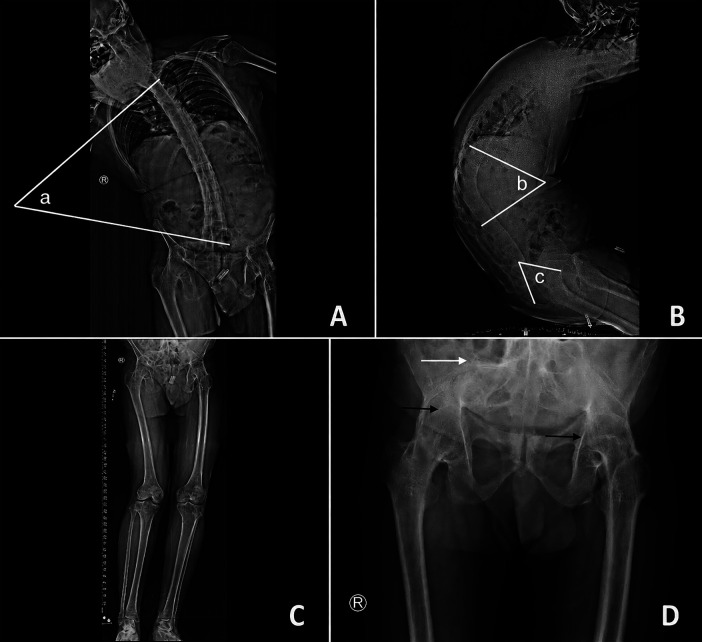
Preoperative x-ray films showed (A) bamboo-like changes in the vertebral bodies in the coronal plane of the spine, with the spine bending to the right, cobb angle of a = 50°; (B) kyphosis in the sagittal plane of the spine, cobb angle of b = 55°, PI of c = 55°; (C) full-length standing lower limb radiographs showing bilateral hip ankylosis, with both hip and knee joints in flexion; (D) bilateral hip orthopantomogram showing disappearance of the sacroiliac joint space and bony fusion (white arrow), both hip joints demonstrate total bony ankylosis (black arrows).
